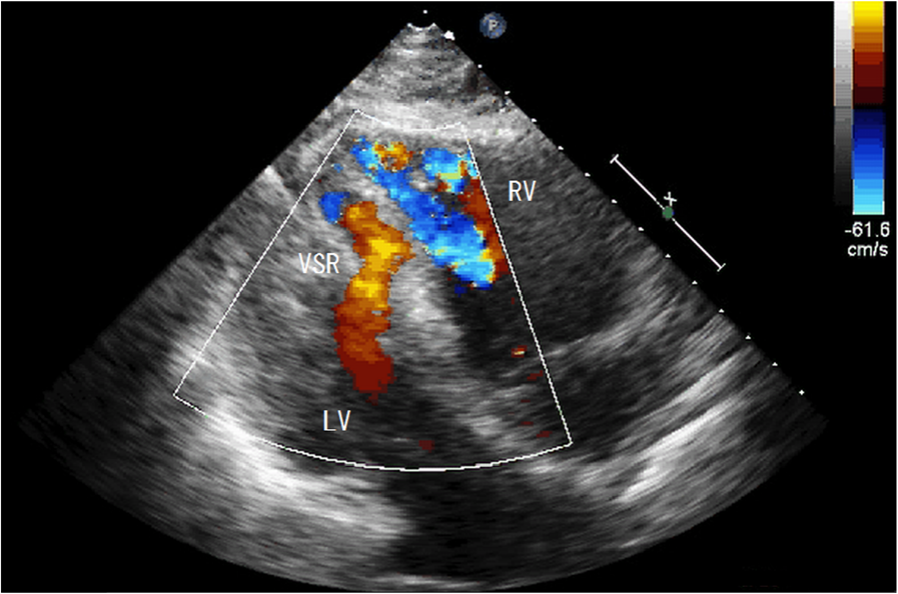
Color Doppler showing left-to-right shunt
